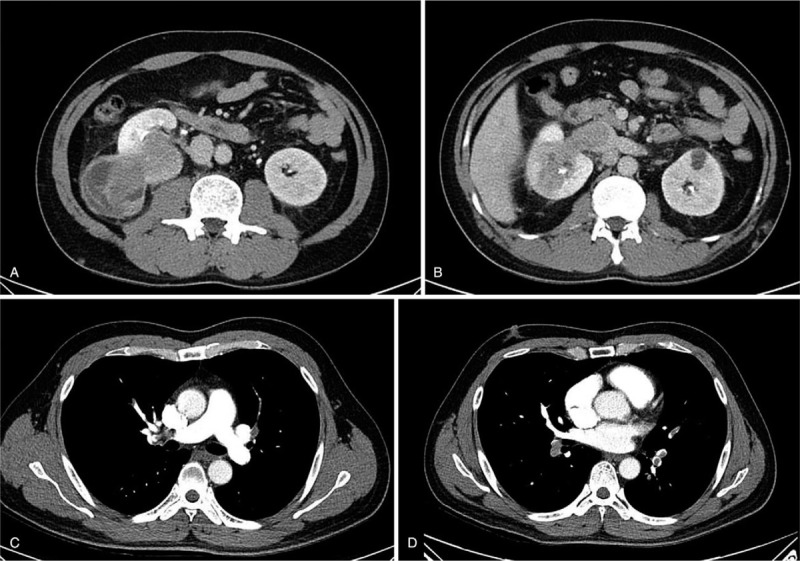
(a) Preoperative abdominal computed tomography scans showing a right renal mass (b) protruding into the inferior vena cava . (c) Preoperative chest computed tomography scans showing pulmonary tumor emboli in the right main pulmonary artery (d) involving both the pulmonary arteries.
